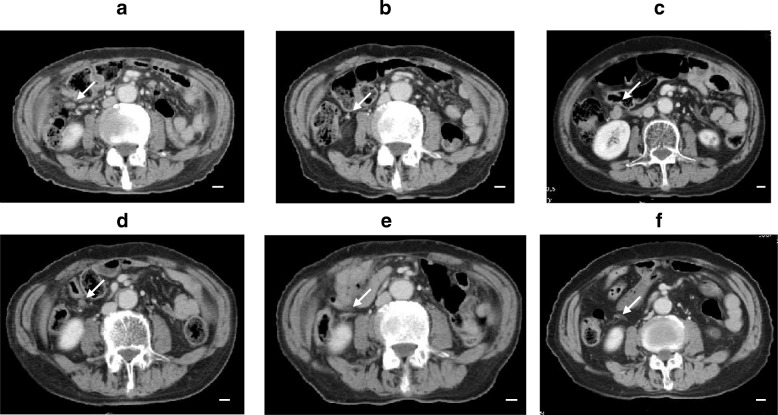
Trastuzumab is effective against HER2-positive gastric cancer. a Abdominal computed tomography (CT) showed a peritoneal nodule (*arrow*). b Paclitaxel was effective against peritoneal dissemination (*arrow*). c CT showed a new peritoneal nodule appeared *(arrow)*. d, e Peritoneal dissemination disappeared (*arrows*). f CT showed peritoneal dissemination was disappeared for 6 years and 3 months after surgery *(arrow)*
